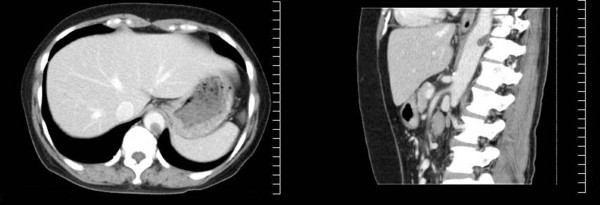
Enhanced computed tomography scan before treatment. A thrombus is observed in the lower-line aortic blood vessel at the level of the diaphragm.
